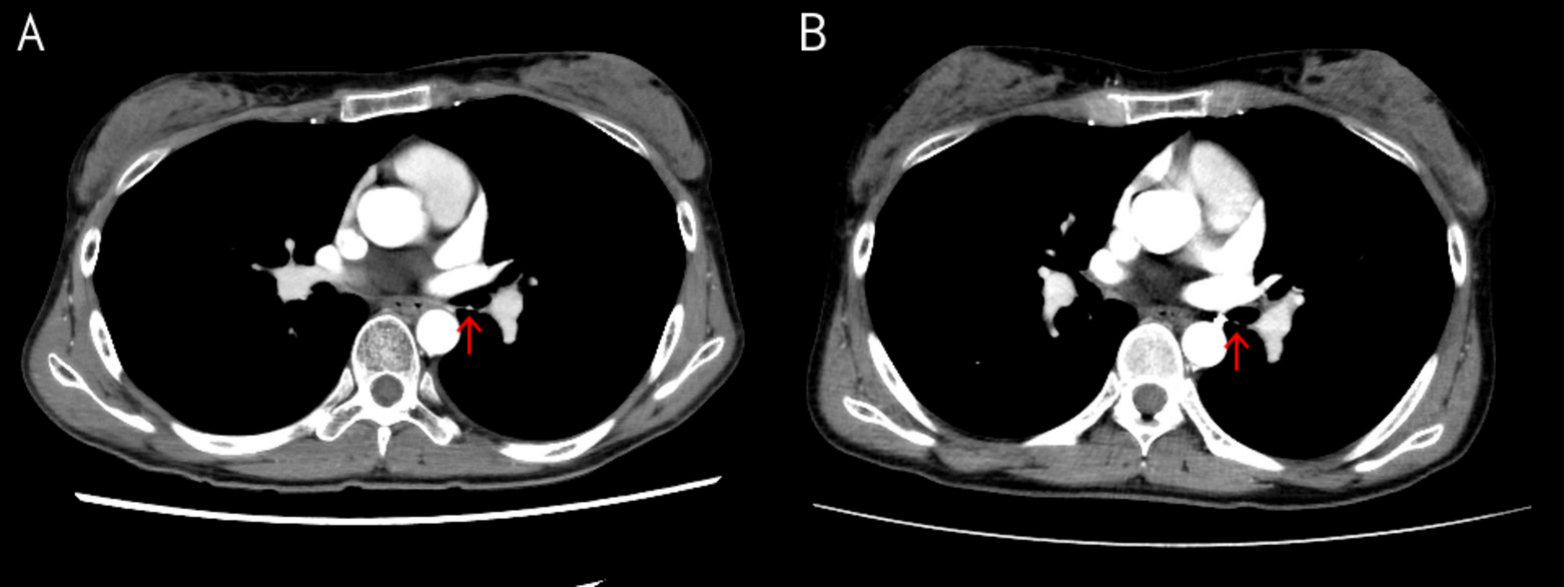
Contrast‐enhanced CT. (A) Endobronchial nodular lesion was enhanced by contrast material. (B) Endobronchial nodular lesion regressed with decreased enhancement after the bronchial artery embolisation.
